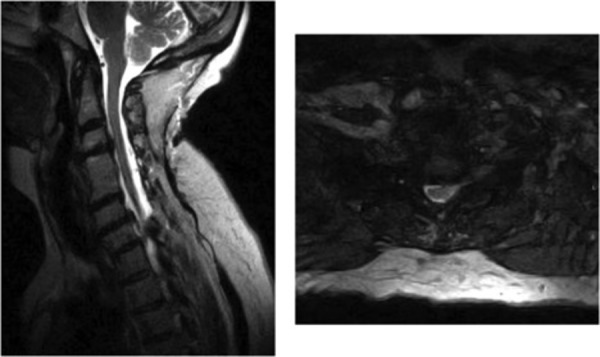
Preoperative sagittal and axial T2 MRI Preoperative sagittal and axial T2 magnetic resonance imaging (MRI) demonstrating bilateral foraminal disease at C5-6 with bilateral foraminal disease and cord impingement at C7 along with severe foraminal disease at this level on the left.
